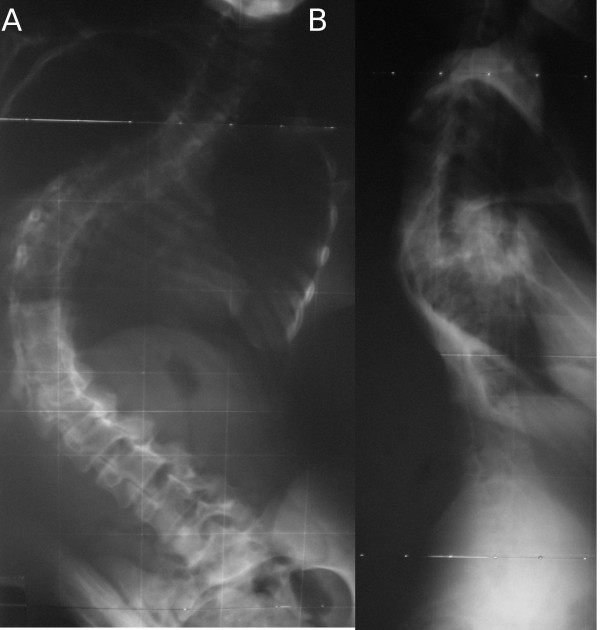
(A, B), Case 2: Spine radiographs at the age of 15; anteroposterior (A) and laterolateral (B) view.
